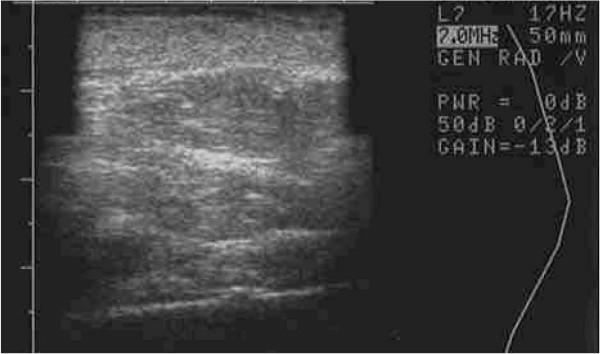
Ultrasound image of left hand (DEP-SVF treated) saggital view of thenar web space of a 69-year-old female 3 months post lipotransfer.
